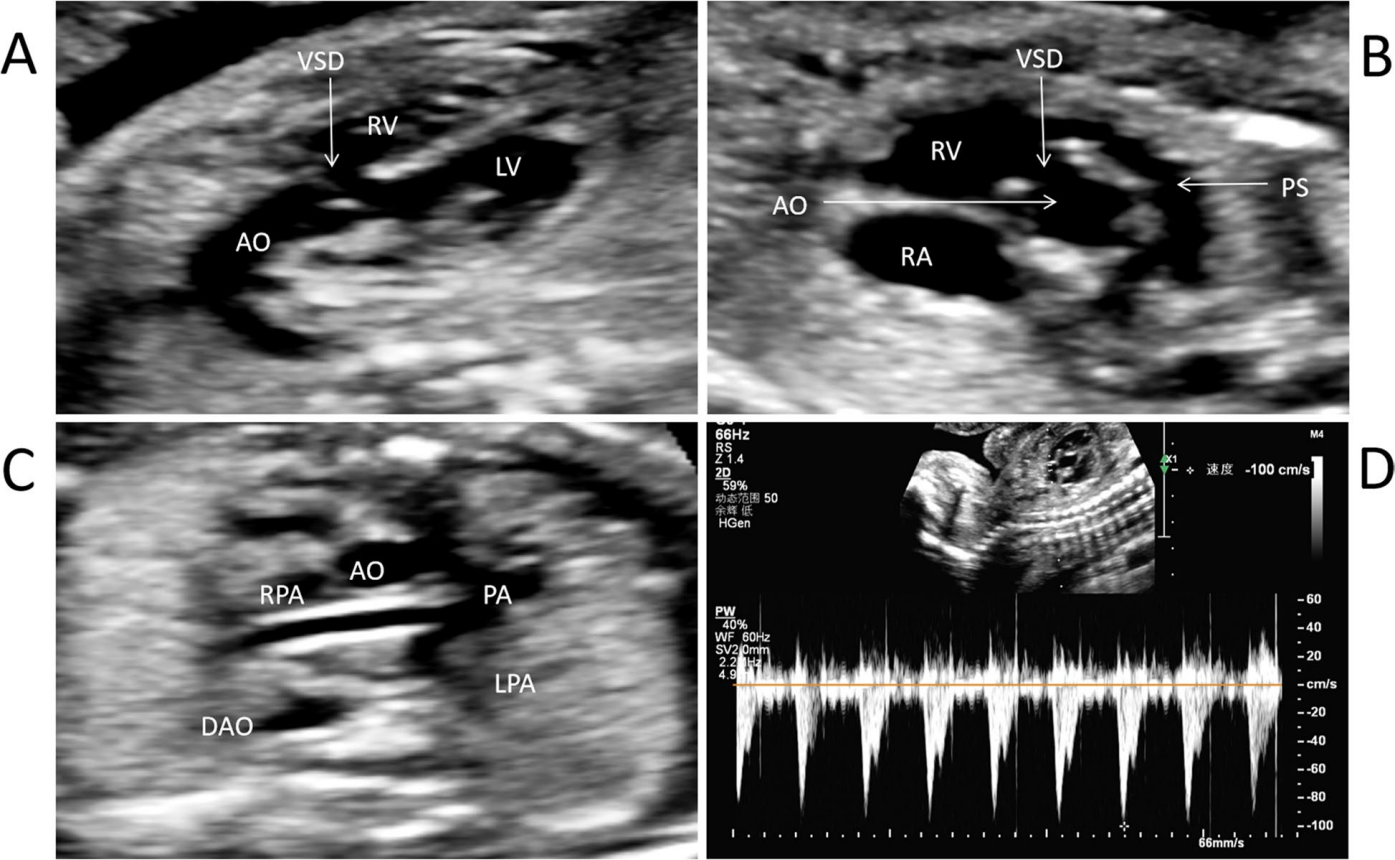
Ultrasonographic observation of fetal TOF. A In the left ventricular outflow tract view, a malalignment-type VSD was detected, and the aorta root overrode the ventricular septum. B In the short axis view of great arteries, the conal septum moved anteriorly, and stenosis of the RVOT and PA were identified. C The diameters of branch pulmonary arteries were slightly small but closed to normal. D The typical “double-peak” pattern in systole of spectral Doppler ultrasound in the PA confirmed stenosis of the PA
